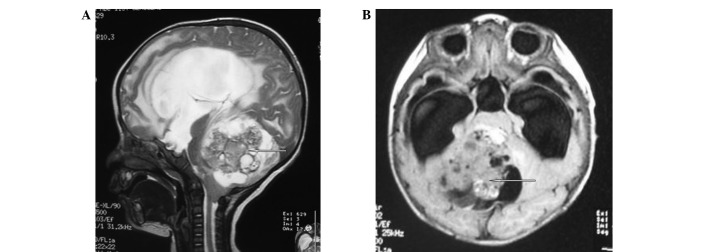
Posterior cranial fossa teratoma (arrowheads) accompanied by hydrocephalus. (A) Sagittal and (B) axial magnetic resonance imaging (MRI) scans.
